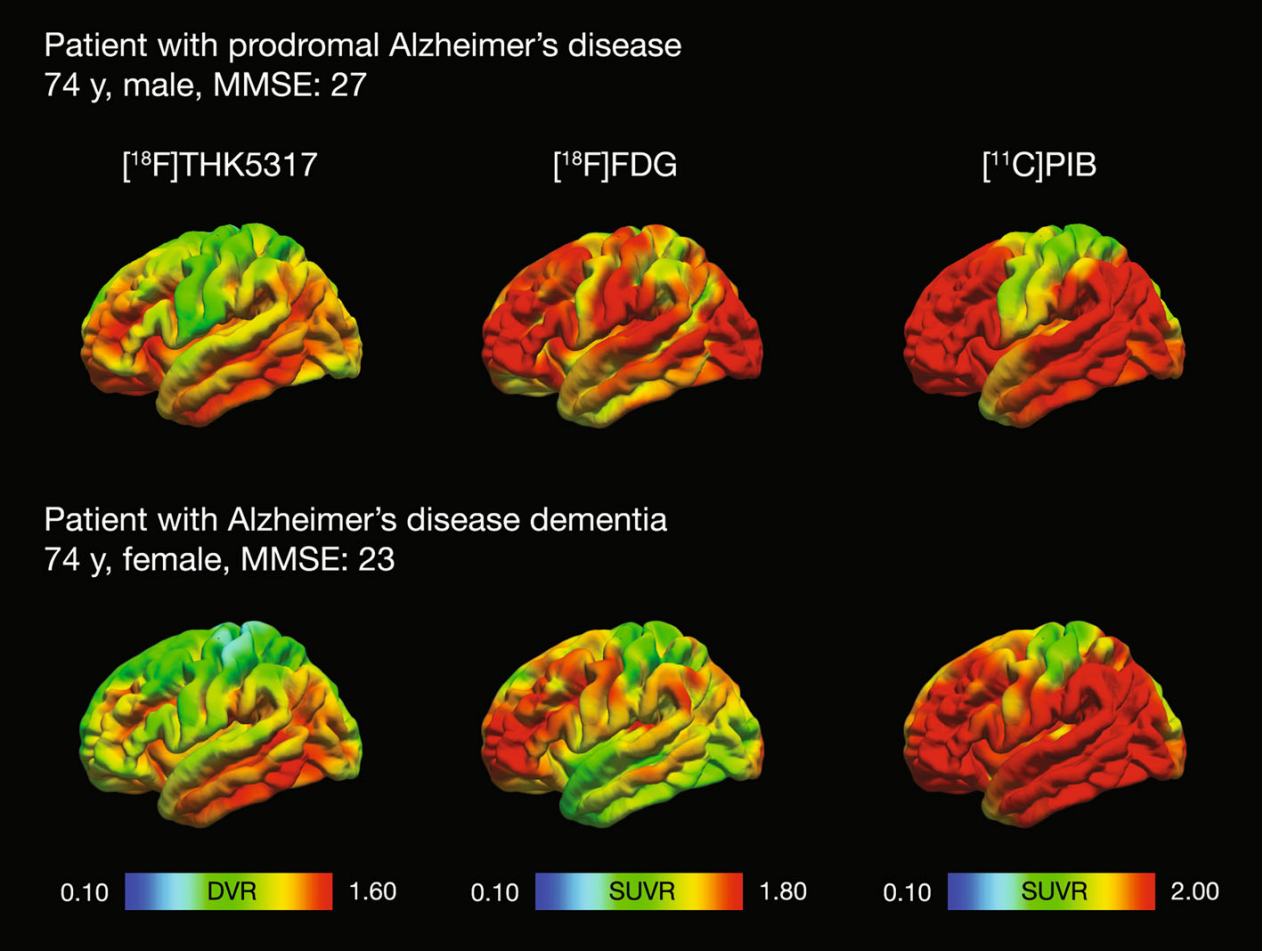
In vivo imaging of AD biomarkers in a patient with prodromal AD and in a patient with AD dementia. The retention of [^18^F]THK5317 and [^11^C]PIB are expressed with reference to the retention in the grey matter of the cerebellum; [^18^F]FDG uptake is expressed with reference to uptake in the pons. AD = Alzheimer’s disease; DVR = distribution volume ratio; FDG = fluorodeoxyglucose; PIB = Pittsburgh compound B; SUVR = standardized uptake value ratio
